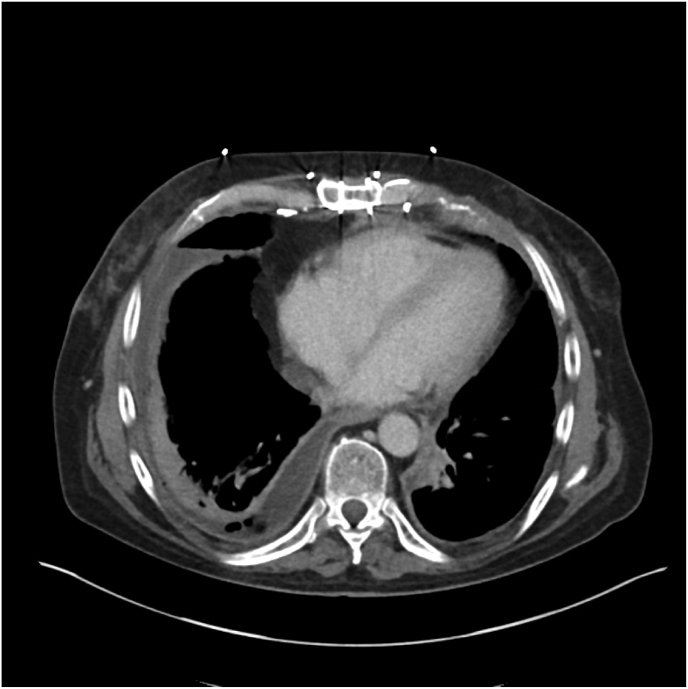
CT-thorax with contrast showing a moderate, right-sided, rim-enhancing hydropneumothorax.
